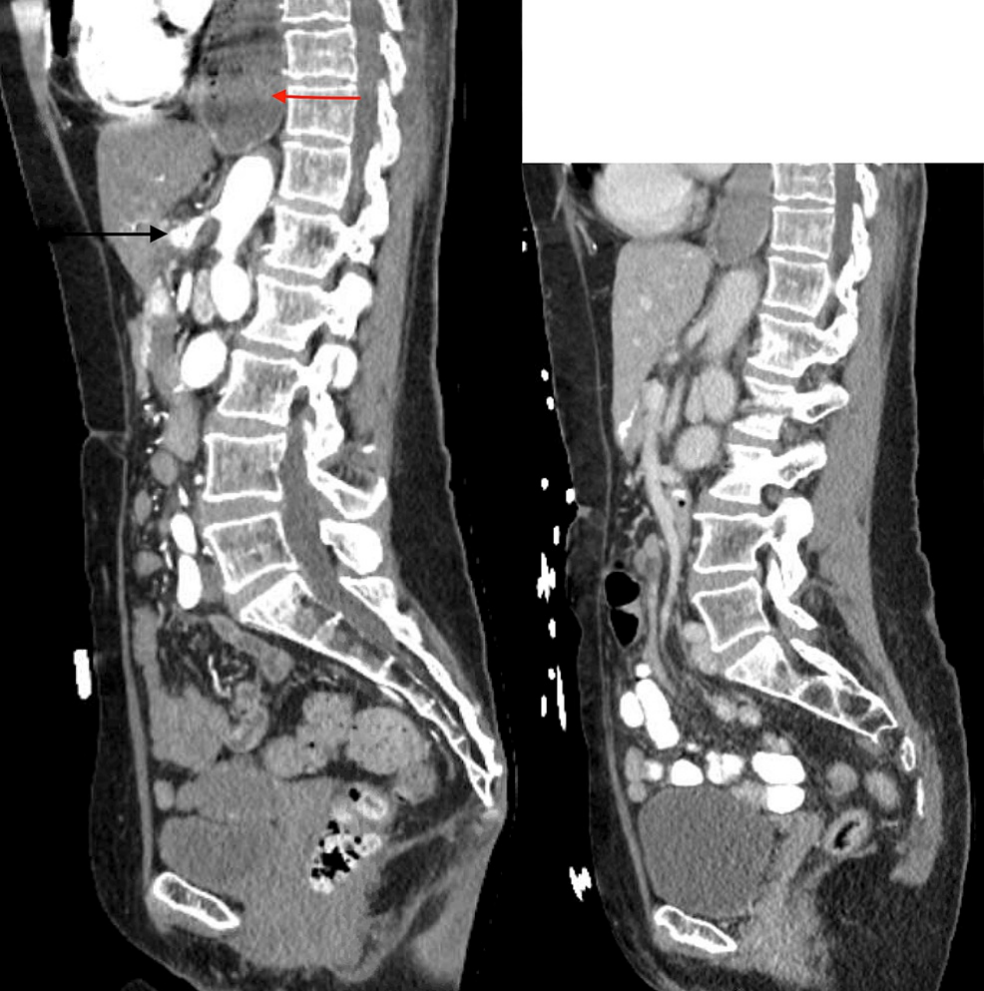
Mid-sagittal computed tomography with intravenous contrast on readmission with abdominal pain (left image) compared to the initial admission computed tomography (right image) The computed tomography scan on the left shows an eight-millimeter celiac artery pseudoaneurysm (black arrow) and a dilated debris-filled esophagus consistent with her known history of achalasia (red arrow). This is new compared to the computed tomography done three days before the onset of her abdominal pain.
